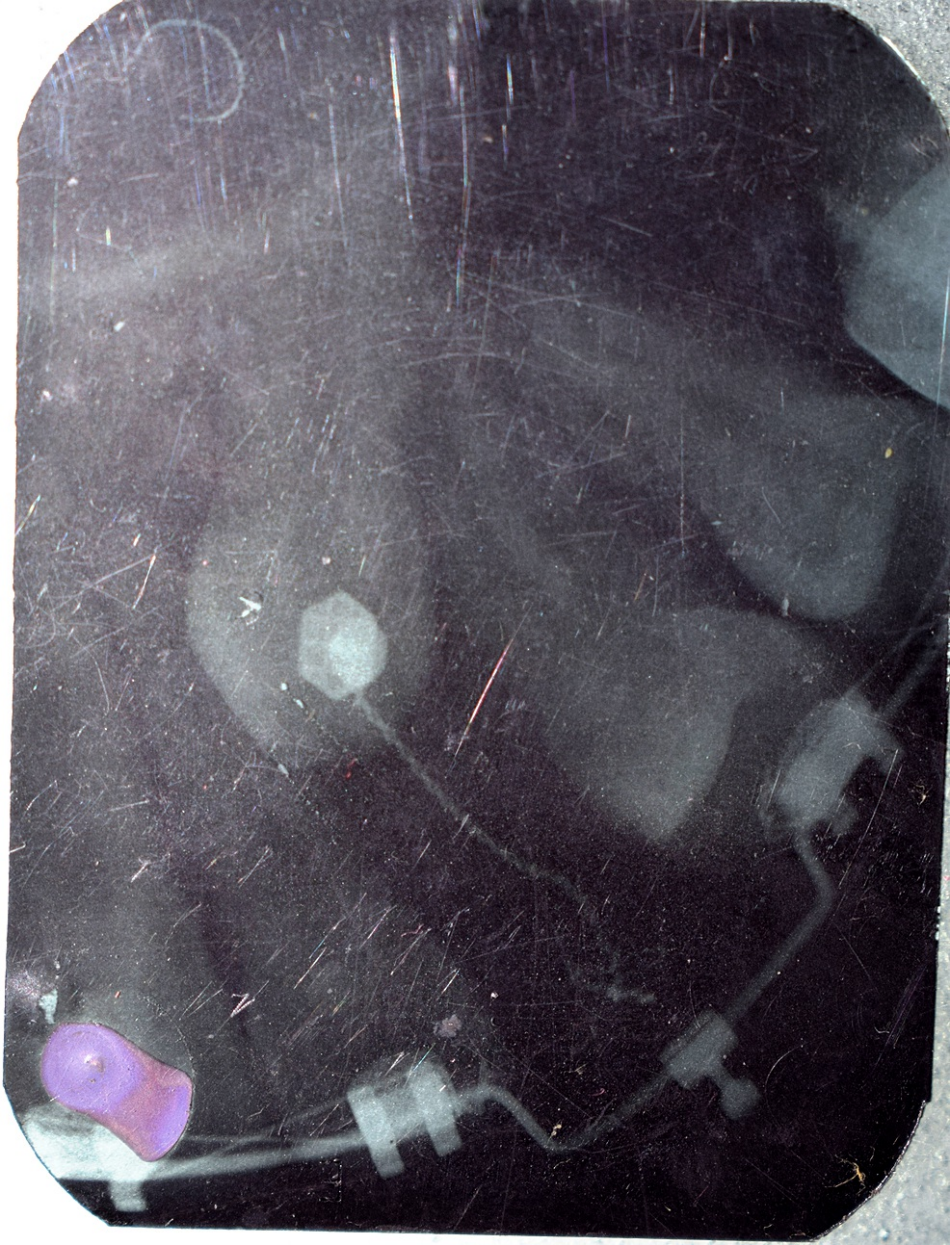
IOPA radiograph showing attachment position IOPA: intra-oral peri-apical
